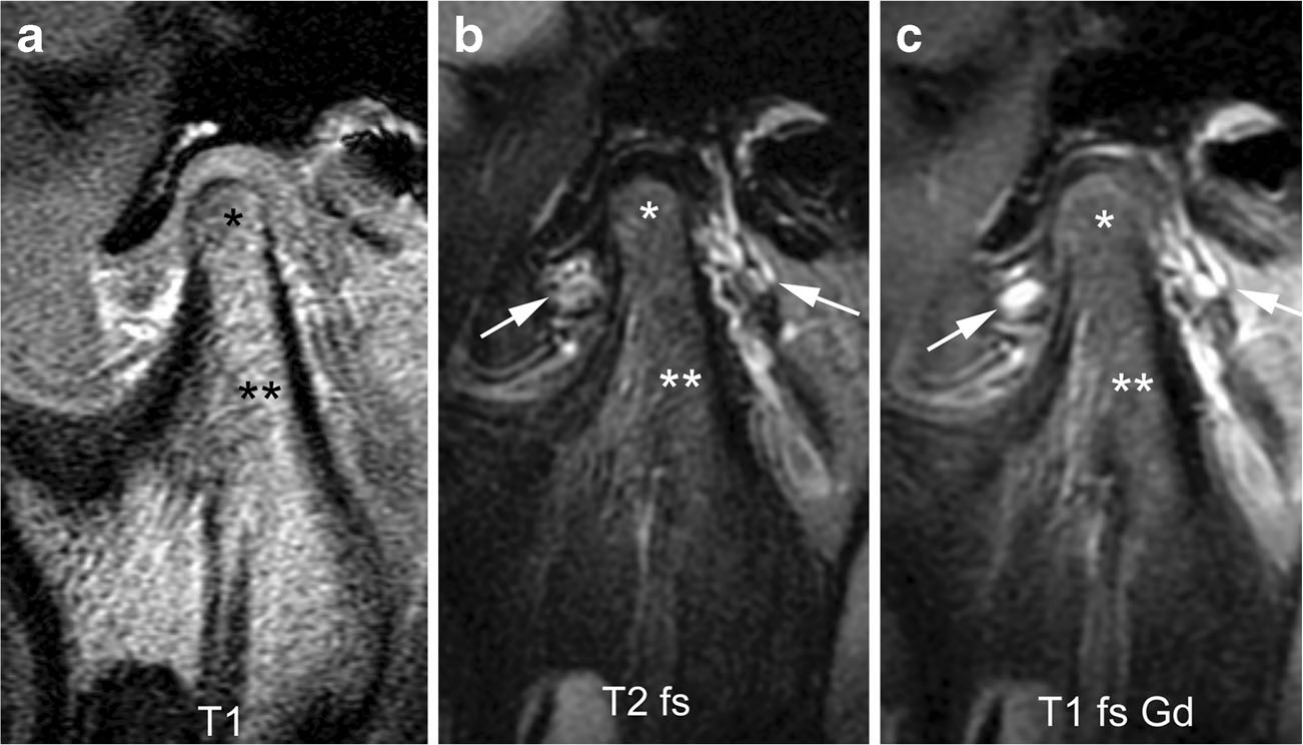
Normal bone marrow signal and enhancement (grade 0) in a 14-year-old boy. a-c Sagittal oblique T1-weighted (TR/TE 300/4.2 ms, flip angle 80°) gradient echo image without fat saturation (a), T2-weighted (TR/TE 5,400/77 ms) fat saturated image (b) and postcontrast fat-saturated T1-weighted (TR/TE 670/10 ms) fast spin echo images (c) show the marrow space of the mandibular condyle (*) with isointense signal compared to that of the mandibular ramus (). Note prominent veins surrounding the joint (arrows in b,c) and slightly increased enhancement of the posterior upper joint compartment. For this age, the condyle appears rather round and lacks an anterior tilt of the mandibular neck. T1 T1-weighted, T1 fs Gd contrast-enhanced fat saturated T1-weighted, T2 fs fat saturated T2-weighted
